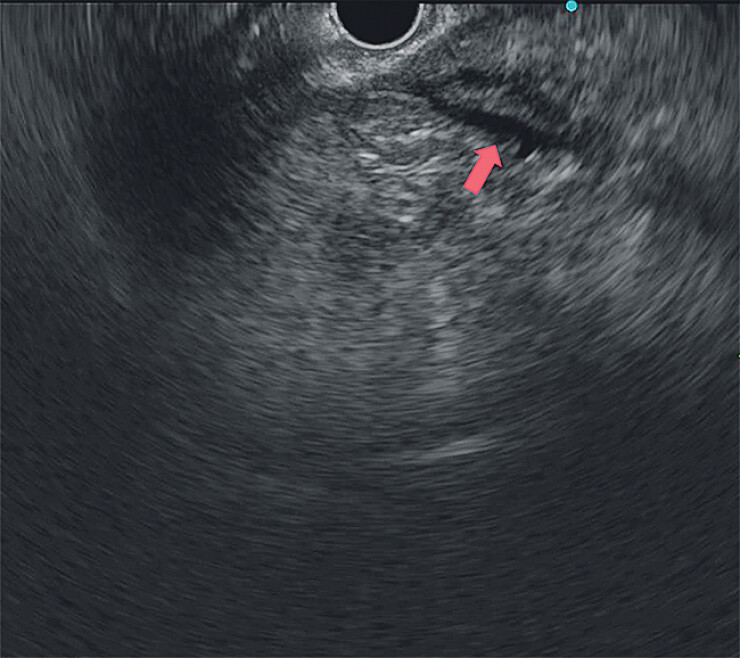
Ultrasound endoscopic image. After foreign body removal (the red arrow points to the main pancreatic duct).
